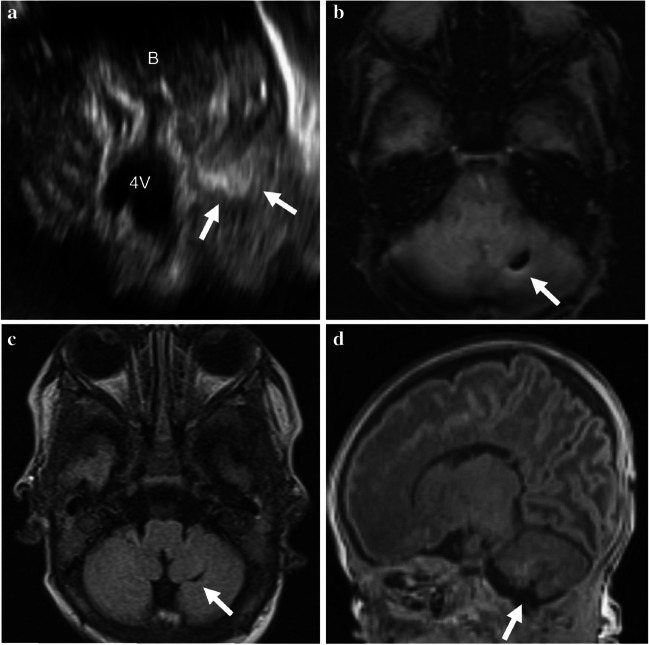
Representative case of type 2 cerebellar hemorrhage in a preterm girl (27 + 4 weeks, 980 g). a Axial right mastoid view of cranial ultrasound image (rotated 90° anticlockwise to align with the axial magnetic resonance image) shows an echogenic lesion (*arrows*) in the left inferior cerebellar cortex. b Axial susceptibility-weighted magnetic resonance image shows a corresponding dark signal lesion (*arrow*). c, d Unenhanced axial (c) and sagittal (d) T1-weighted magnetic resonance images show focal volume loss in the left inferior peripheral cerebellar cortex (*arrows*). B brain stem, 4 V fourth ventricle
